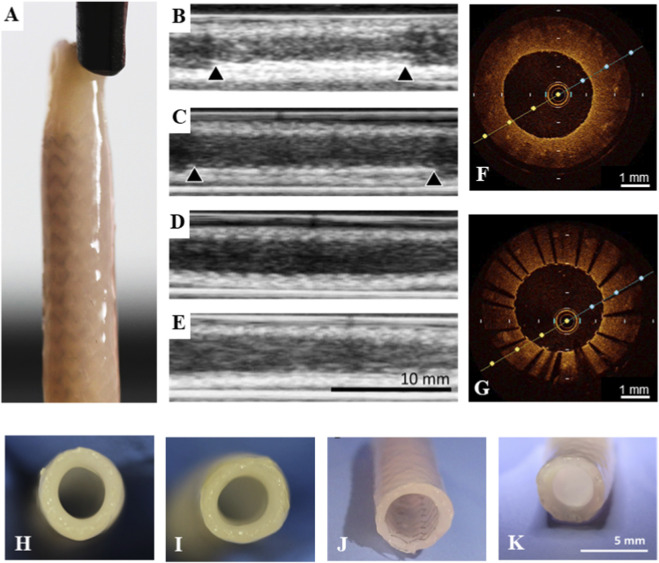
POBA and stent implantation in TEBVs (A) TEBV with a commercially available human-sized coronary stent. (B–E) Ultrasound monitoring proved useful for non-invasive monitoring after implantation of BMS (B) and DES (C), after POBA (D), and in untreated control TEBVs (E). Boundaries of the stents (black arrowheads) as well as the TEBV vessel walls were clearly visible. (F,G) OCT-imaging was performed 14 days after POBA and stent implantation. (H–K) Macroscopic views of control (H), POBA-treated (I), BMS-treated (J), and DES-treated (K) TEBVs.
